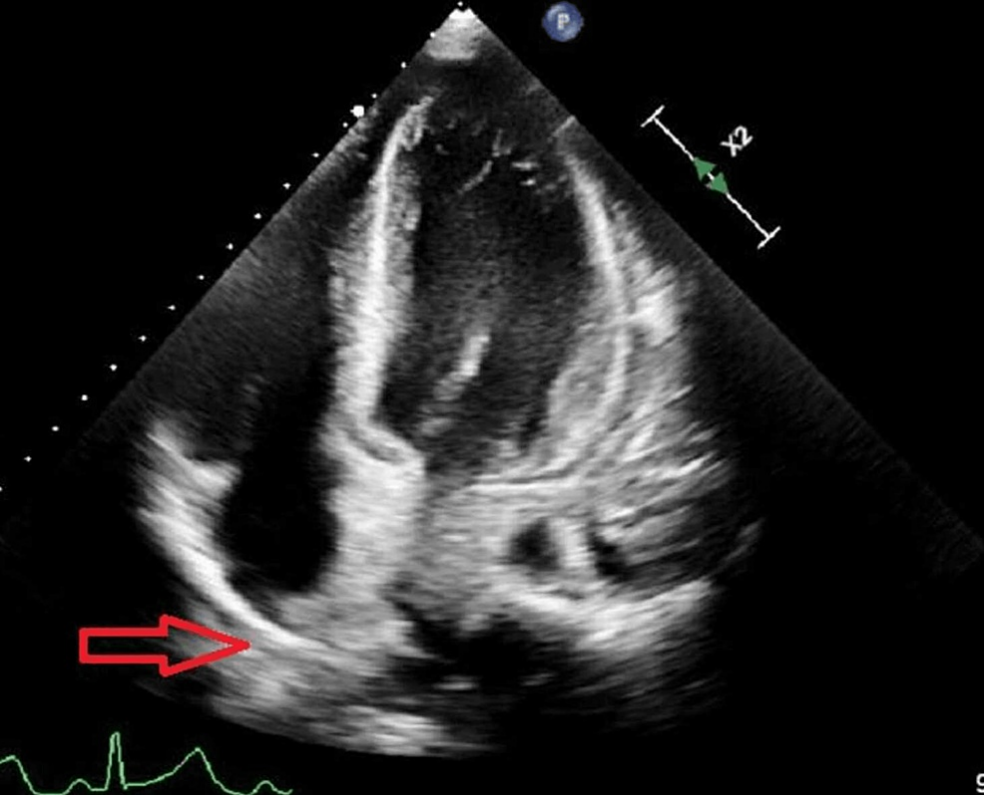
Repeat echocardiography after pericardiocentesis showing resolution of previously observed pericardial effusion. The red arrow indicates the region previously occupied by the pericardial effusion (now no longer present).
